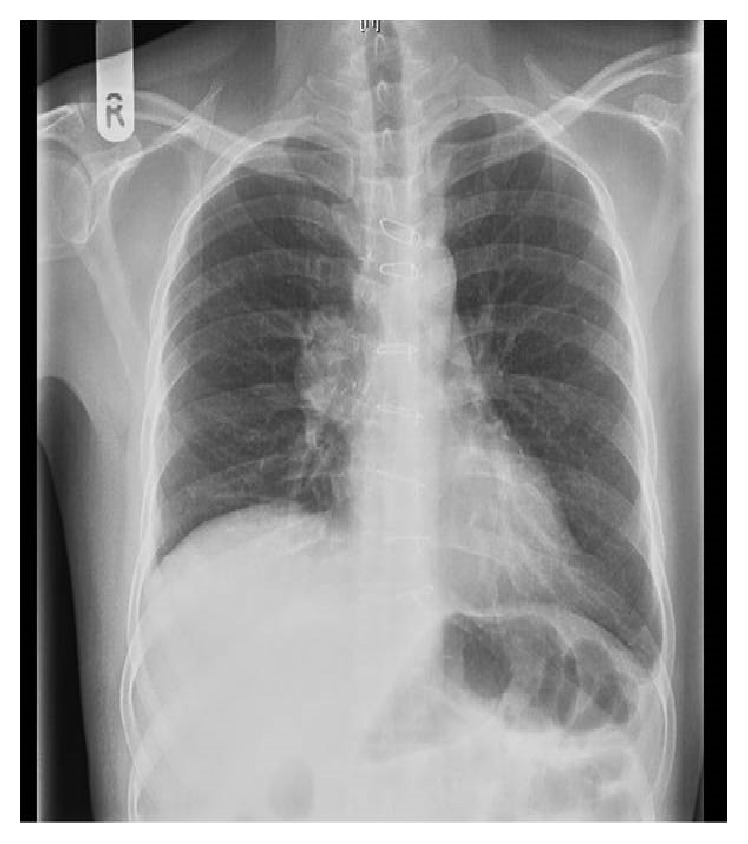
Postoperative chest X-ray showing residual mass in the anterior mediastinum.
